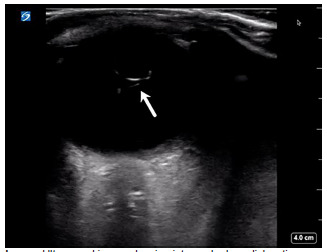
Ultrasound image showing intraocular lens dislocation (arrow) into the posterior chamber.
